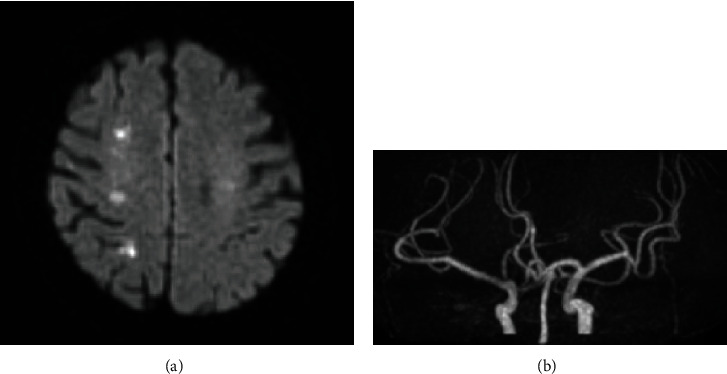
Brain MRI in postoperative day 4. (a) DWI. Multiple high signal intensity in the right cerebrum. (b) Magnetic resonance angiography. No stenosis in the main vessels of the brain.
